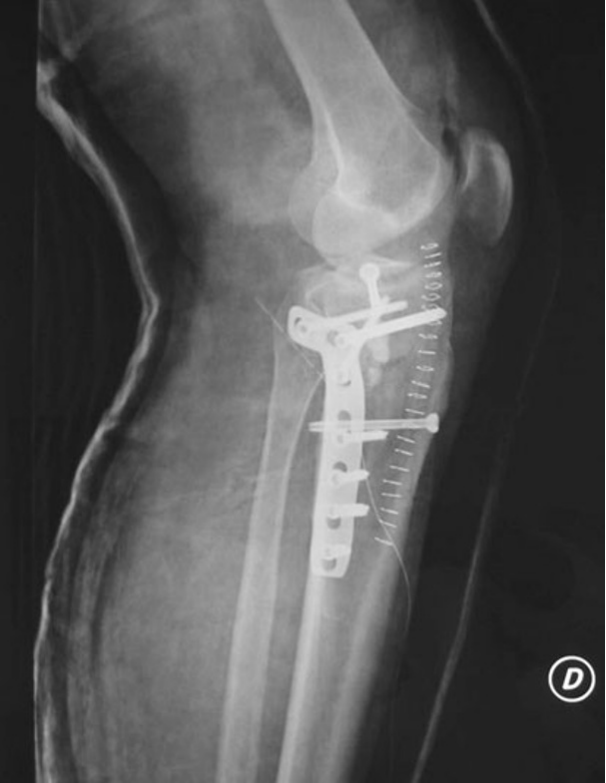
Schatzker type IV fracture, post-op X-rays (LL)
